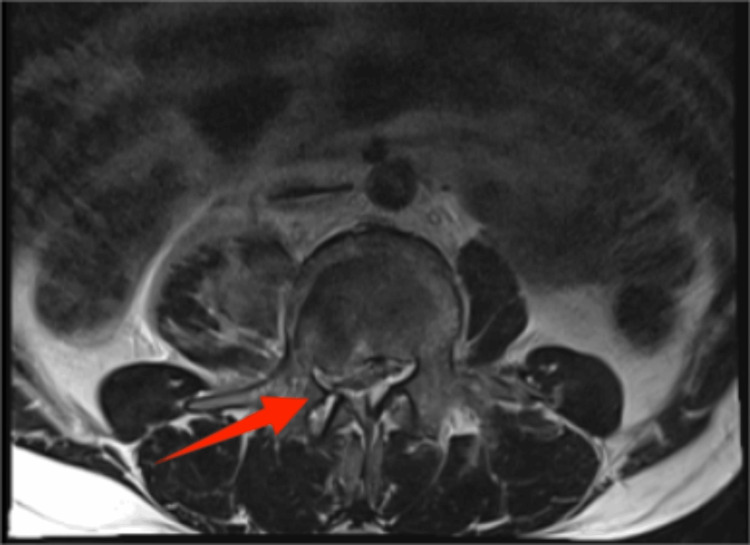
MRI axial image showing L3 vertebral level MRI, magnetic resonance imaging
